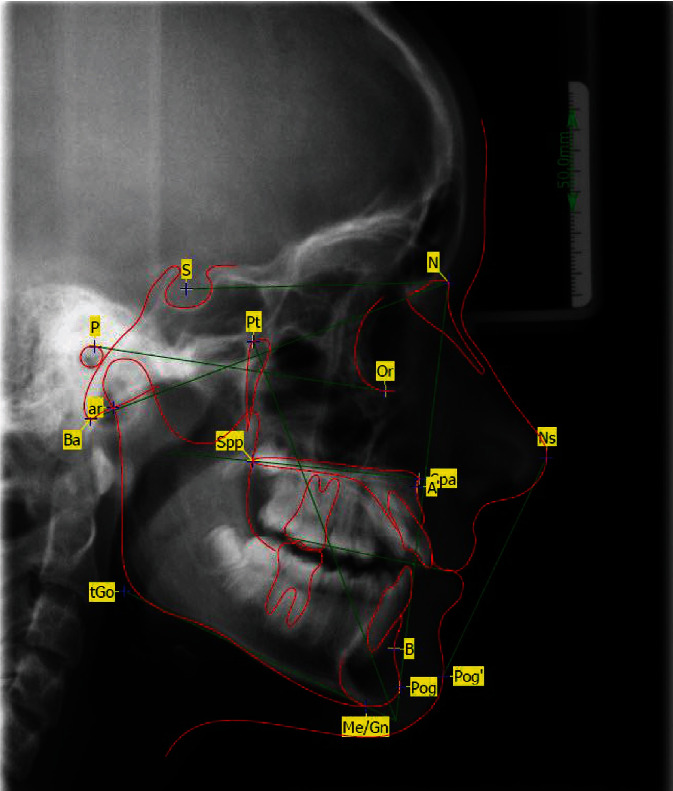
Lateral cephalometric radiograph with evaluation 12 months postoperative; for abbreviations, see also Table 1.
